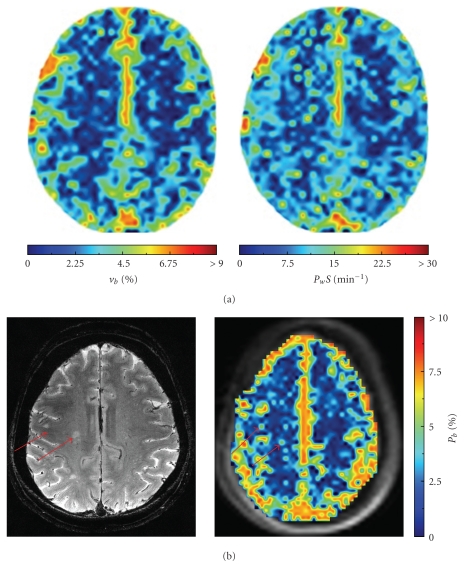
7 T (a) DCE-MRI maps of blood volume (*v*
